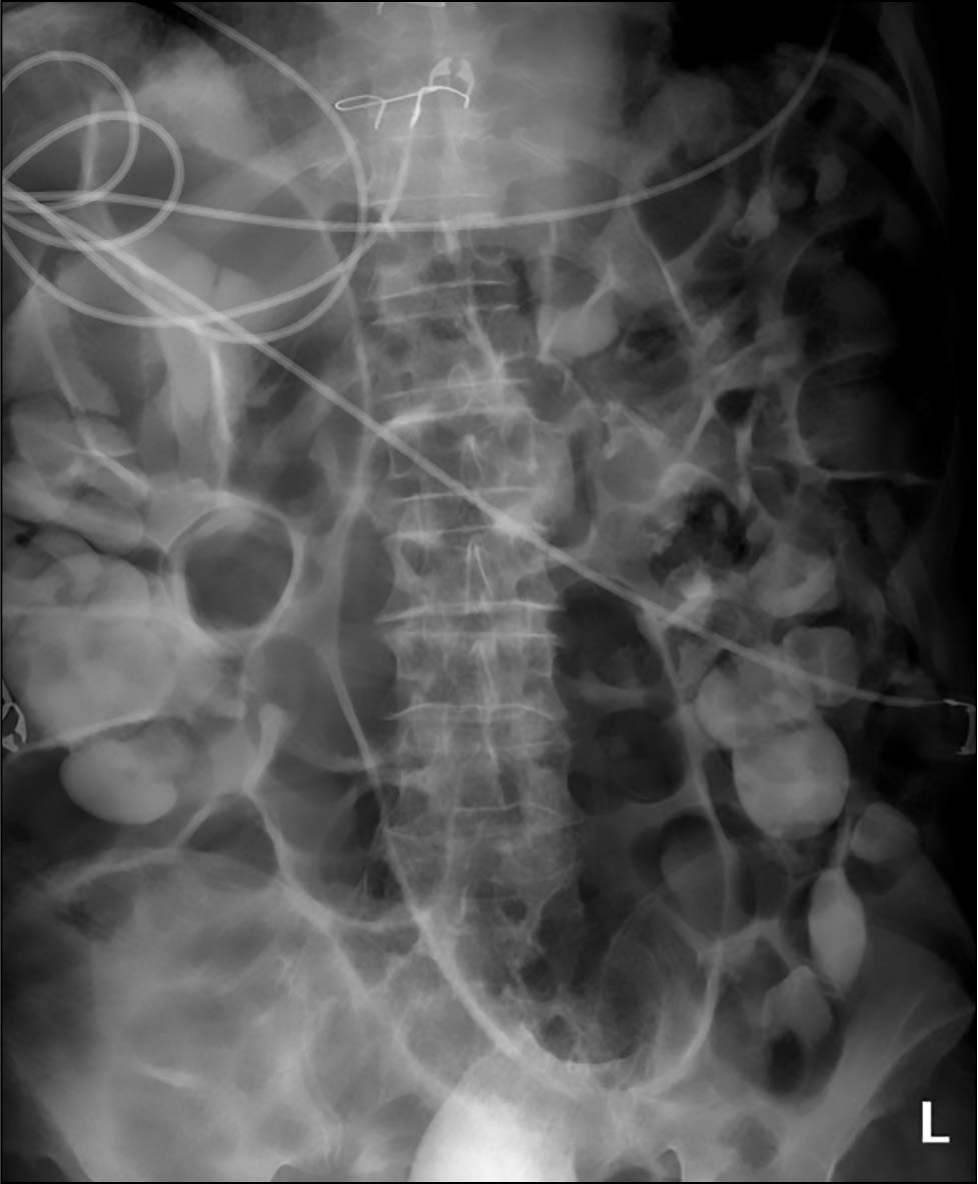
Plain radiograph showing a “coffee bean” sign suggestive of organoaxial sigmoid volvulus.
